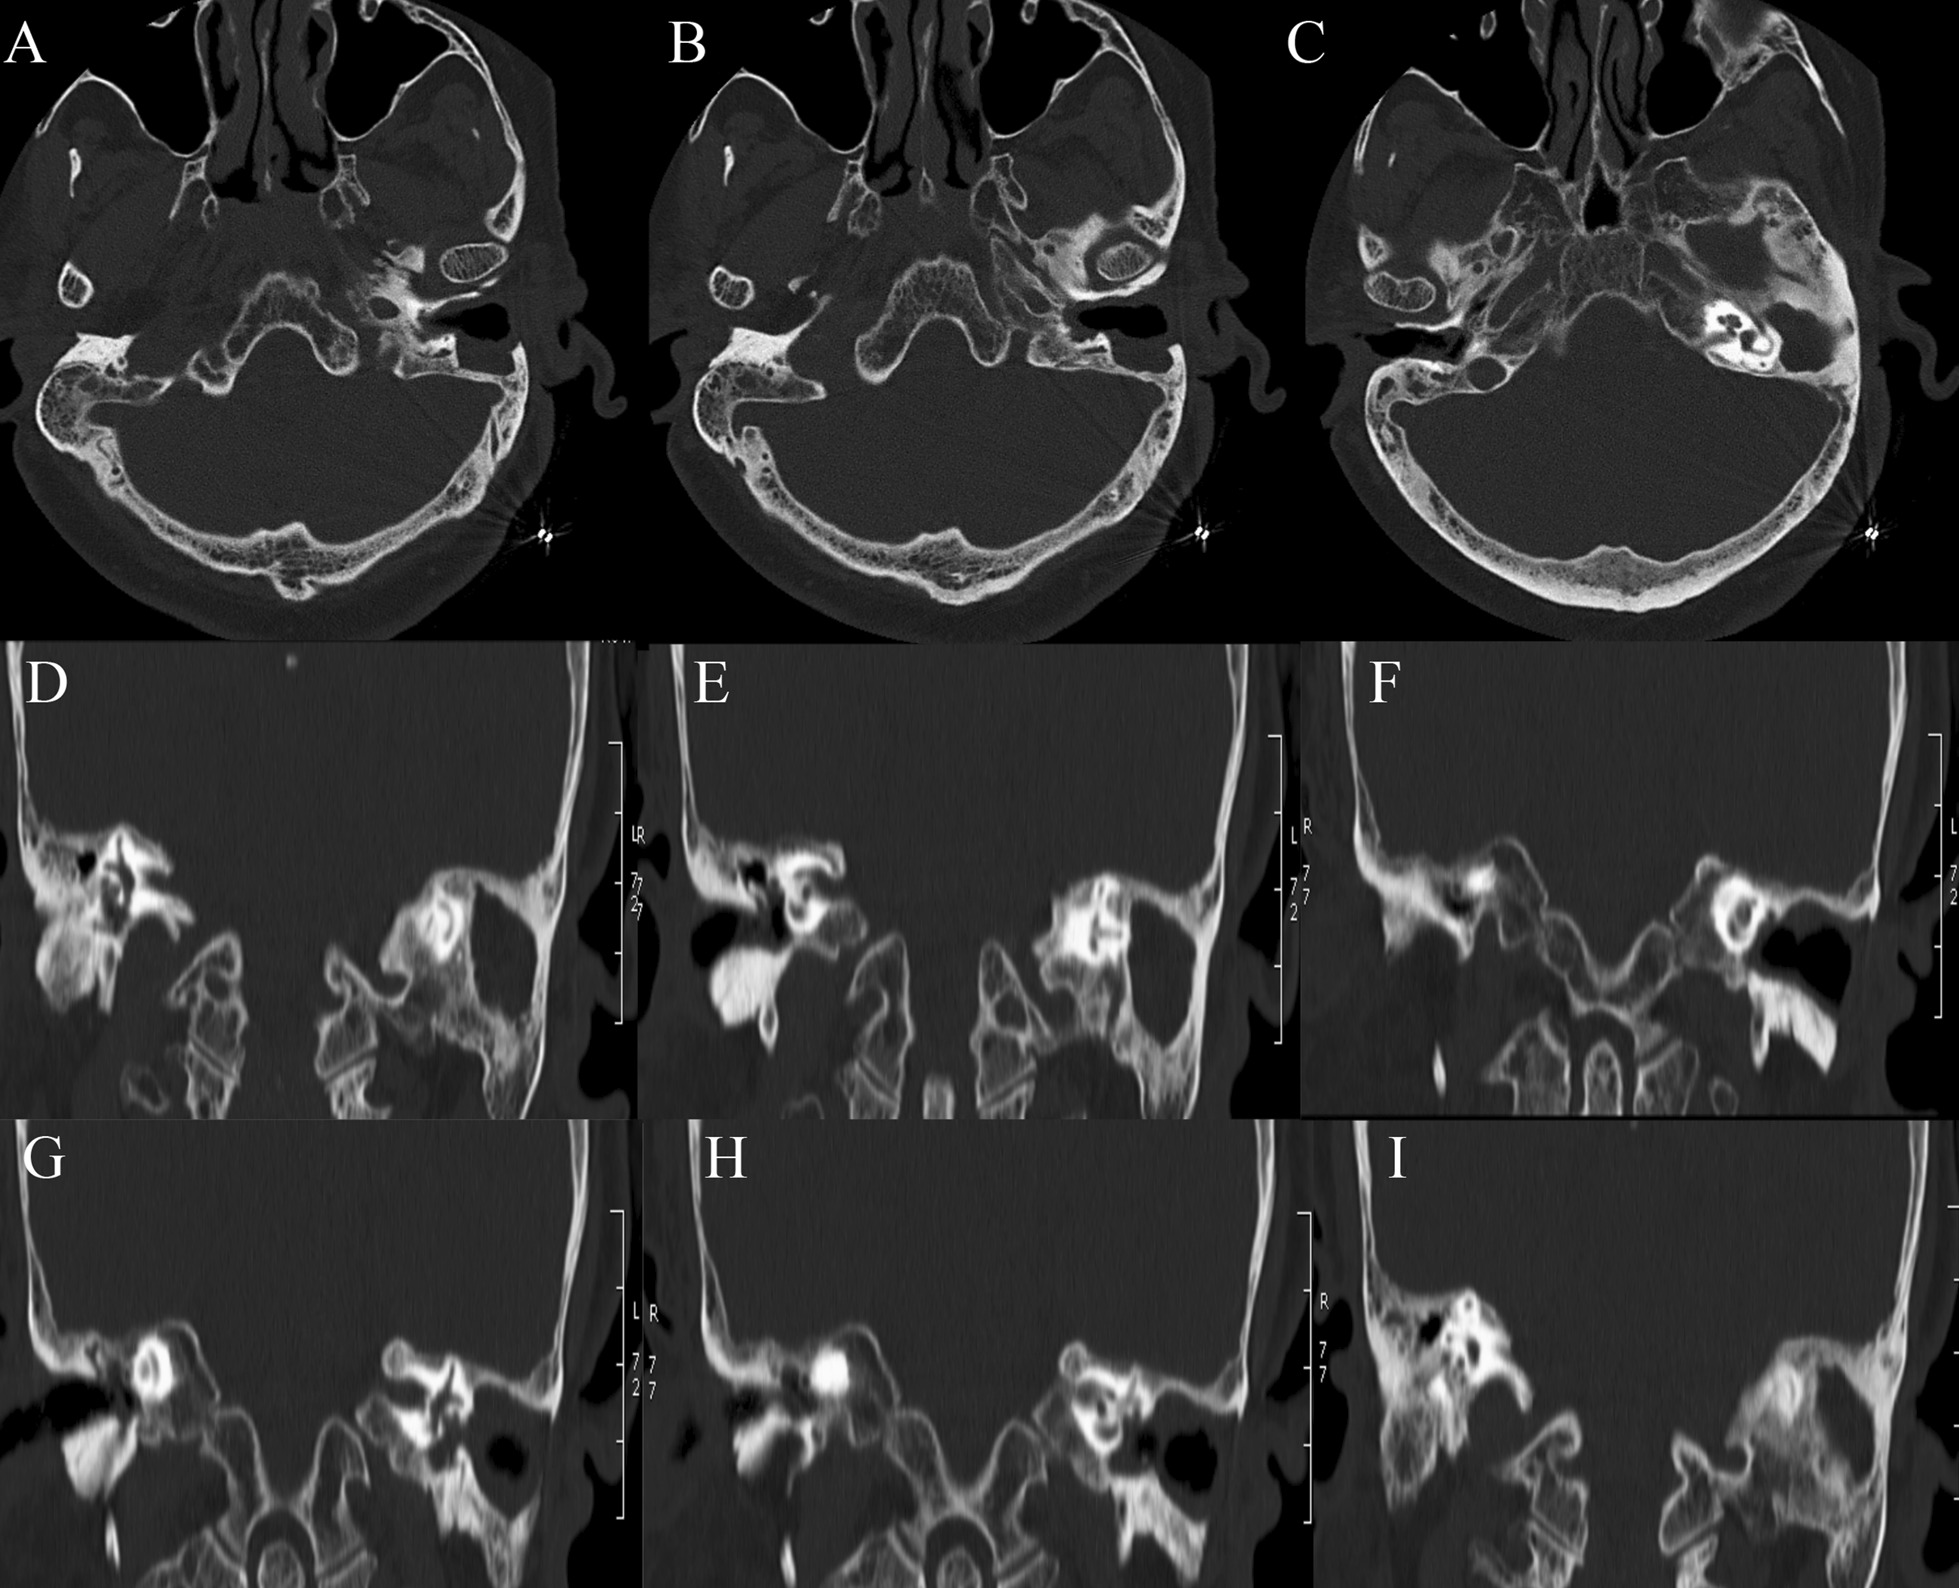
Computed tomography appearance in axial imagines (a, b, c)) and coronal imagines (d, e, f, g, h, i)
